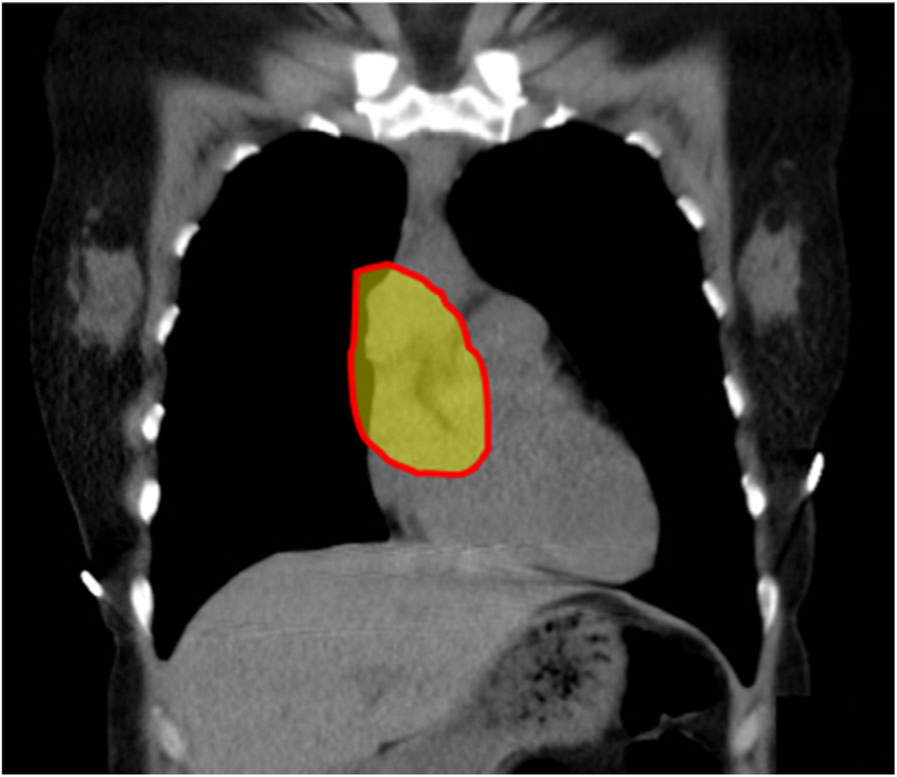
CT scan with highlighted region demonstrating location 4x4cm resection involving pericardium and portion of phrenic nerve
